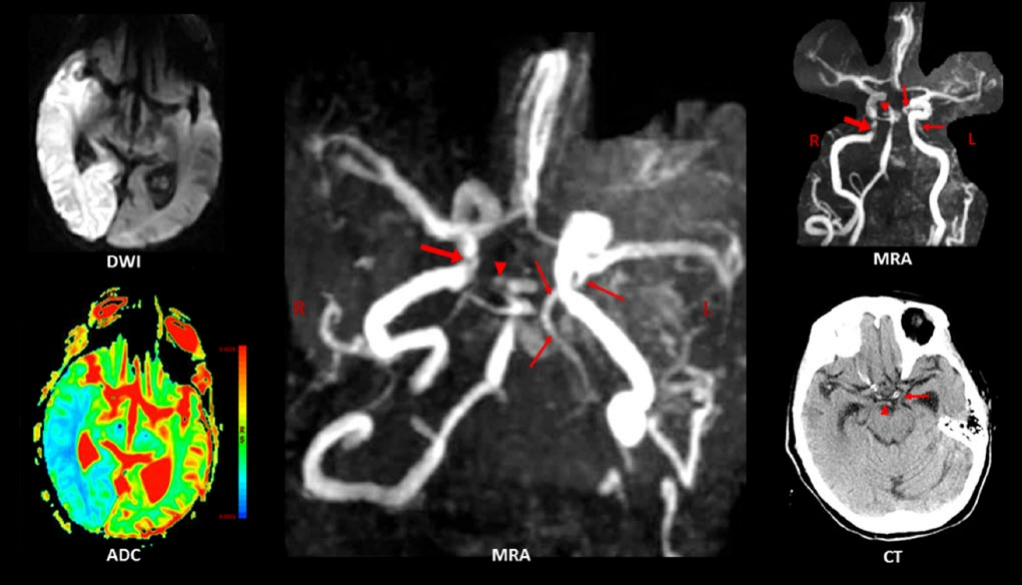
Patient 1 had acute infarcts in the right MCA and PCA territories (hyperintense DWI, low ADC). The 3D time-of-flight (TOF) MRA images show a left FPCA (long arrow), a remnant of the P1 segment (arrowhead) of the right PCA (the rest of it is absent), stenosis at the petrous segment of the right ICA (short arrow), stenosis of the basilar artery above and below the SCA origin (only right SCA is visible), and absent flow in the left vertebral artery. The CT scan image shown was taken 2½ years prior to admission and retrieved from her old records. It clearly shows an intact classic right PCA (arrowhead) and a left fetal-type PCA (long arrow).
